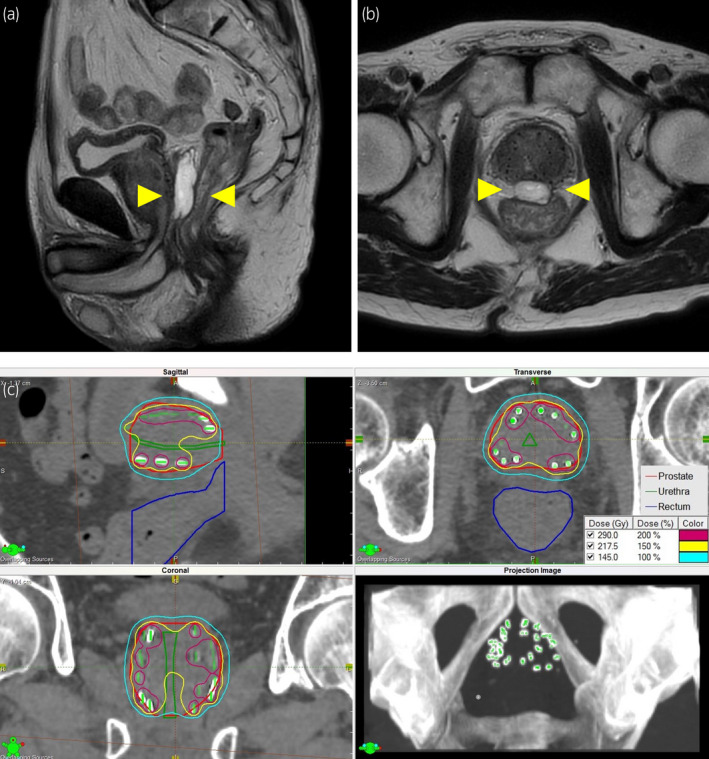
(a, b) Sagittal and transverse views of magnetic resonance imaging immediately after brachytherapy. The spacer (yellow arrowheads) was properly injected between the prostate and the rectum. (c) Isodose curve evaluation of prostate and rectum. The prostate is contoured in red, with 100% and 150% isodose lines in yellow and light blue, respectively. The rectum is contoured in blue. [Colour figure can be viewed at wileyonlinelibrary.com]
